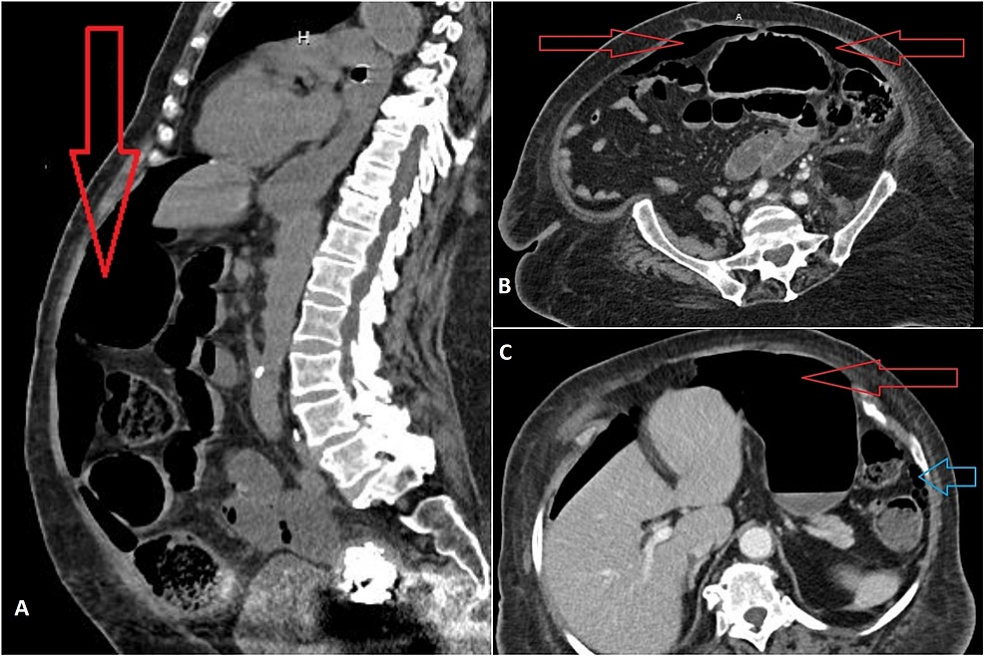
Abdominopelvic CT scan A: sagittal view. B and C: axial views. Red arrows indicate pneumoperitoneum while the blue arrow indicates gas in the pericolic fat suggestive of colonic perforation CT: computed tomography
